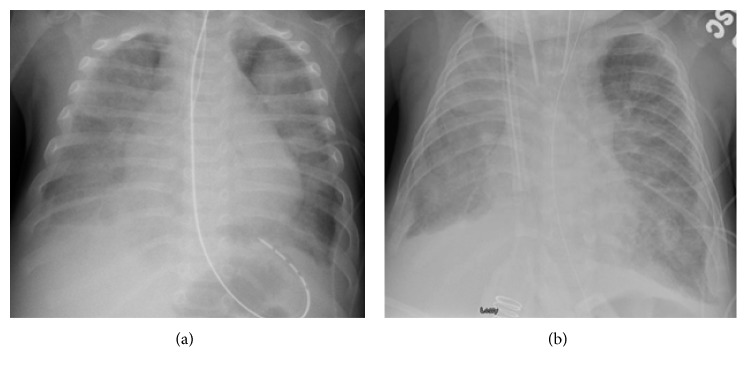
(a) Chest X-ray on admission showing bilateral pulmonary edema with pleural effusions. (b) Pleural effusion unresponsive to therapy with diuretics (hospital day #6).
